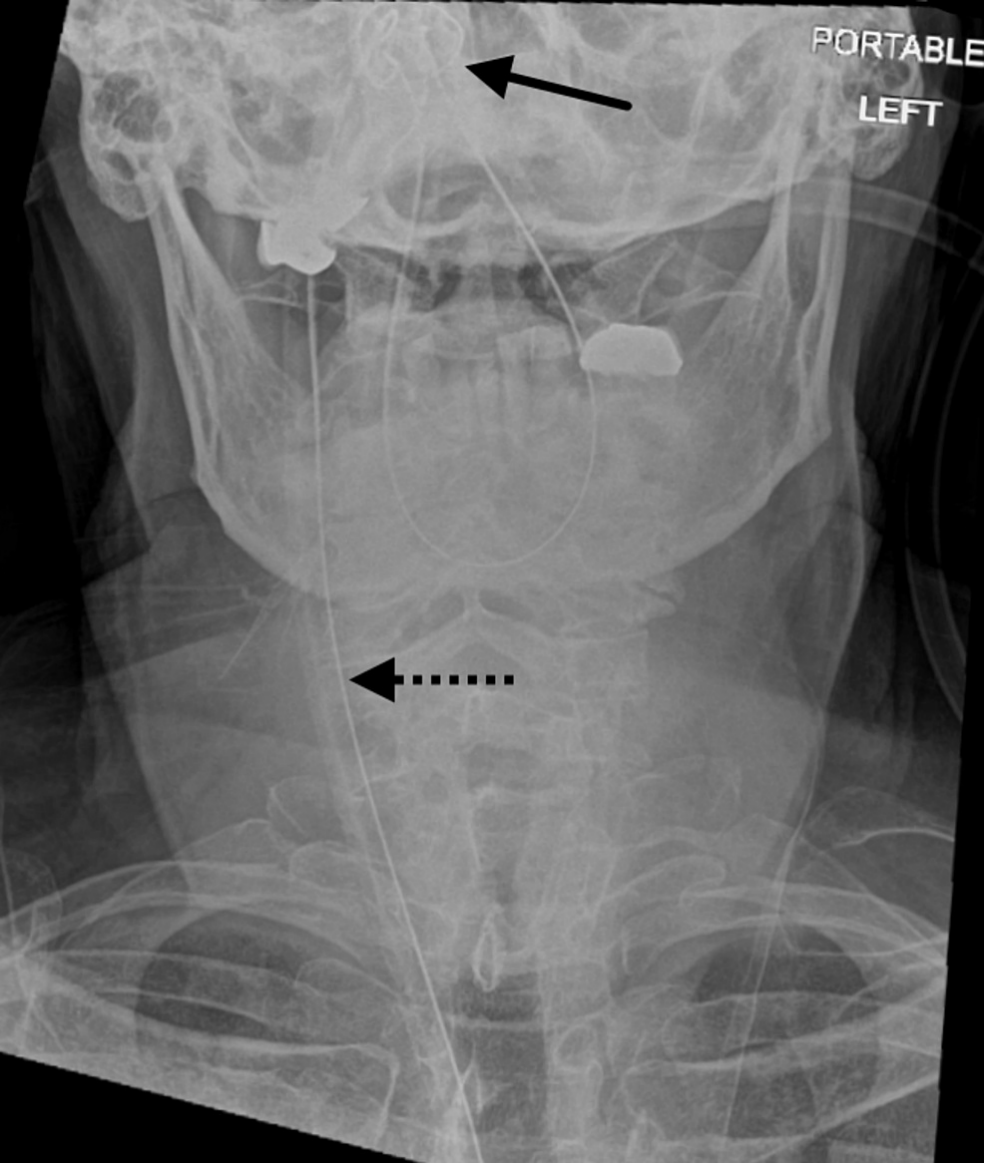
X-ray of a knotted nasogastric (NG) tube in the patient's pharynx. The solid arrow indicates the knot in the NG tube, while the dotted arrow points to the proximal NG tube located outside the body.
